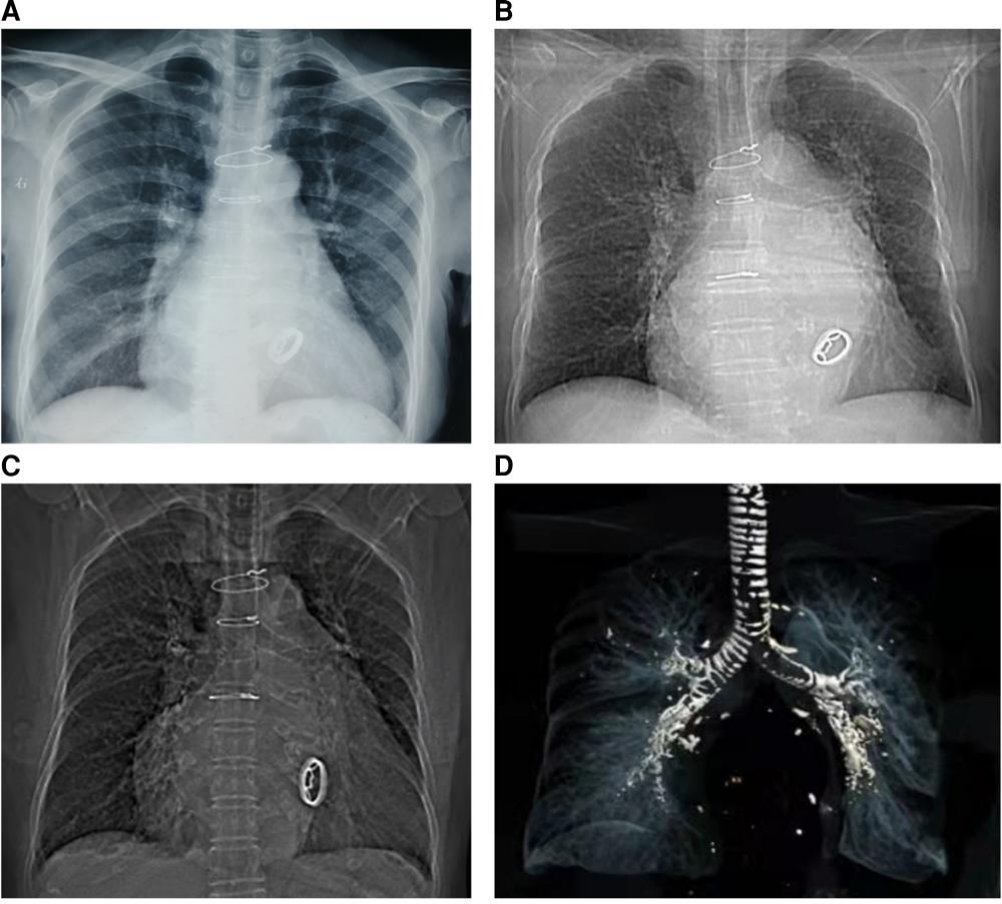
A series of chest X-ray indicated the progressive calcification in the tracheobronchial tree. (*A*) Sixteen years after mitral valve replacement, the chest radiography was unremarkable. (*B*) Ten years prior to admission, the chest radiography revealed a mild diffused tracheobronchial calcification. (*C*) Chest imaging at admission, 31 years after mitral valve replacement showed severe tracheobronchial calcification. (*D*) The remoulding of the tracheobronchial tree revealed diffused and severe tracheobronchial tree calcification.
